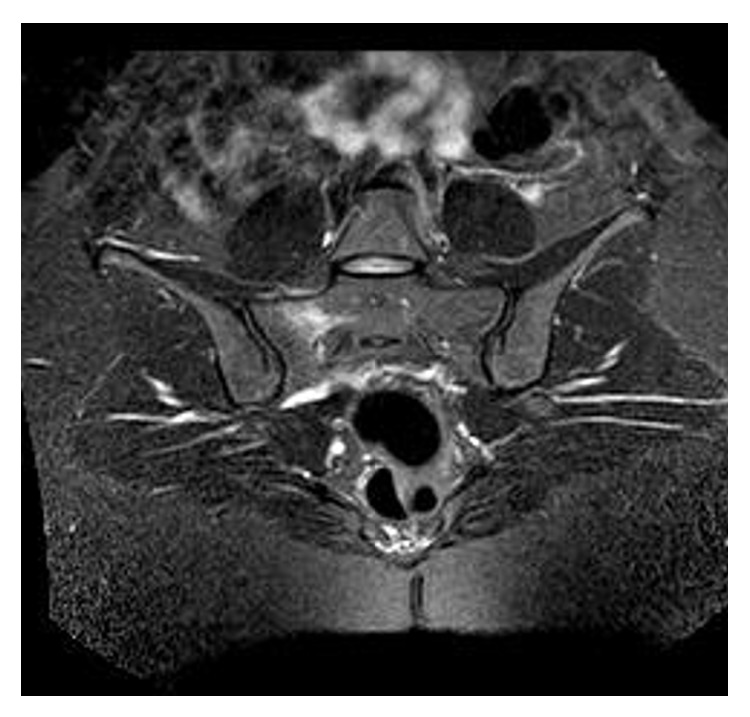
MRI T2 axial scan showed bone marrow edema at the sacrum.
